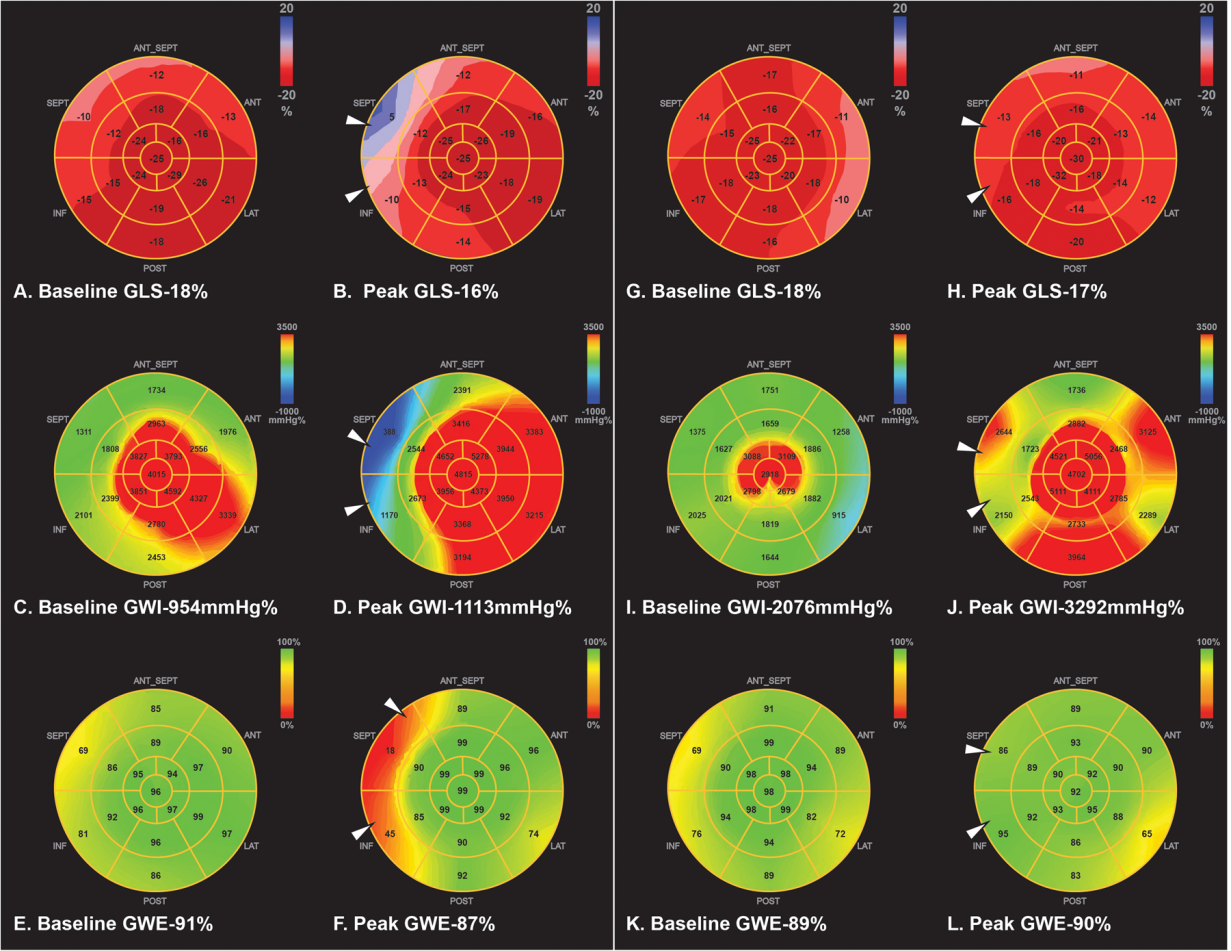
Example of two patients with hypertensive response during DSE. First patient was a 56 y/o female that had basal inferior and inferior septum wall motion abnormality at peak stress. Peak SBP was 262/78 mmHg. GLS and regional longitudinal strain were decreased at peak stress in the RCA territory compared to rest (A,B). GWI was increased from rest to peak stress, but regional MW was deceased in the RCA territory (C,D). Peak GWE and regional MW efficiency was also decreased in the RCA territory (E,F). Cardiac catheterization showed total occlusion of the mid RCA with collaterals to the distal RCA. Second patient was a 41 y/o male that showed similar wall motion abnormality (basal inferior and inferior septum). Peak SBP was 250/111 mmHg. However, peak GLS and regionals longitudinal strain were unchanged (G,H). GWI and regional MW in the RCA region were increased at peak stress (I,J). GWE and regional MW was preserved at peak stress (K,L). Subsequent cardiac PET was negative for coronary ischemia. DSE, dobutamine stress echocardiogram; GLS, global longitudinal strain; GWI, global myocardial work index; GWE, global myocardial work efficiency; MW, myocardial work; RCA, right coronary artery; PET, positron emission tomography.
